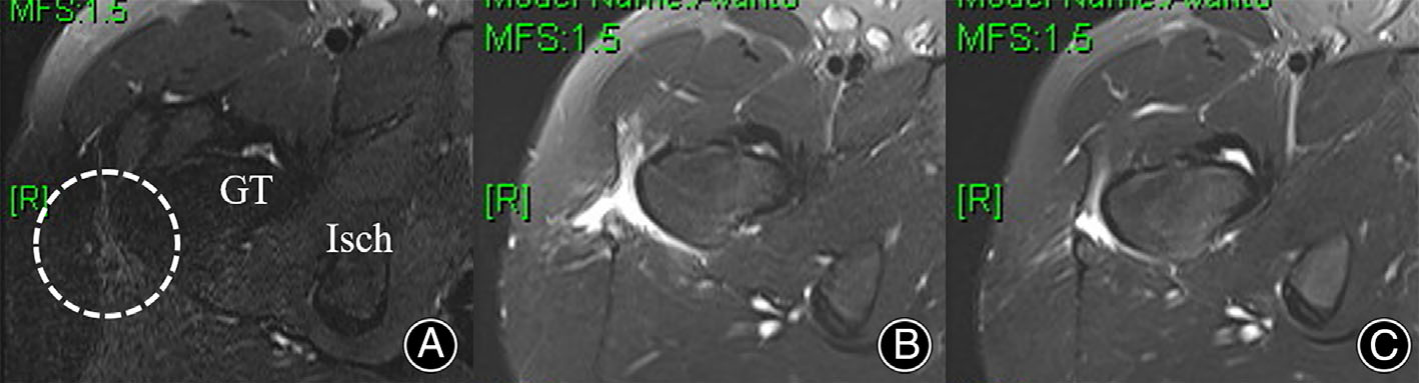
Preoperative axial T2‐weighted magnetic resonance image showing focal thickening and calcification of the anterior edge of the gluteus maximus (A, circle). Postoperative image showing that the lesion involving the GT of the femur has been adequately released (B). Postoperative 3‐month follow‐up image showing adequate healing of the resected area (C). GT, greater trochanter; Isch, ischium.
